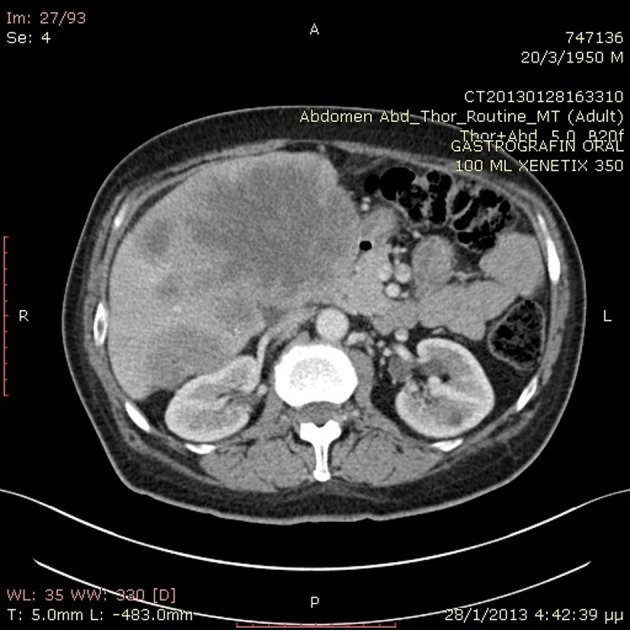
Abdominal CT scan revealed metastatic liver disease.
